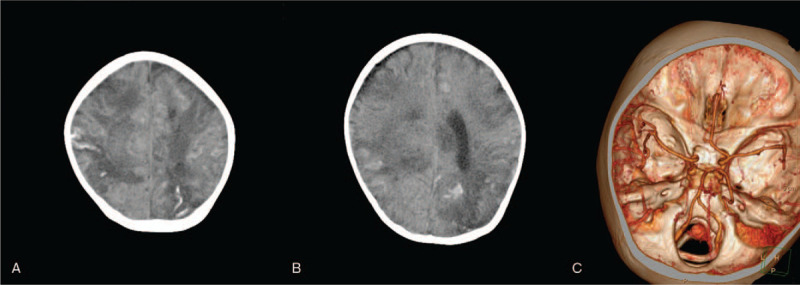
Head CT on day 35. Note: A and E are T1WI, B1 and B2 are T2WI, B and F are FLAIR, C and G are FLAIR, and D and H are DWI. DWI = diffusion-weighted imaging. FLAIR = fluid attenuated inversion recovery, T1WI = T1 weighted image, T2WI = T2 weighted image.
